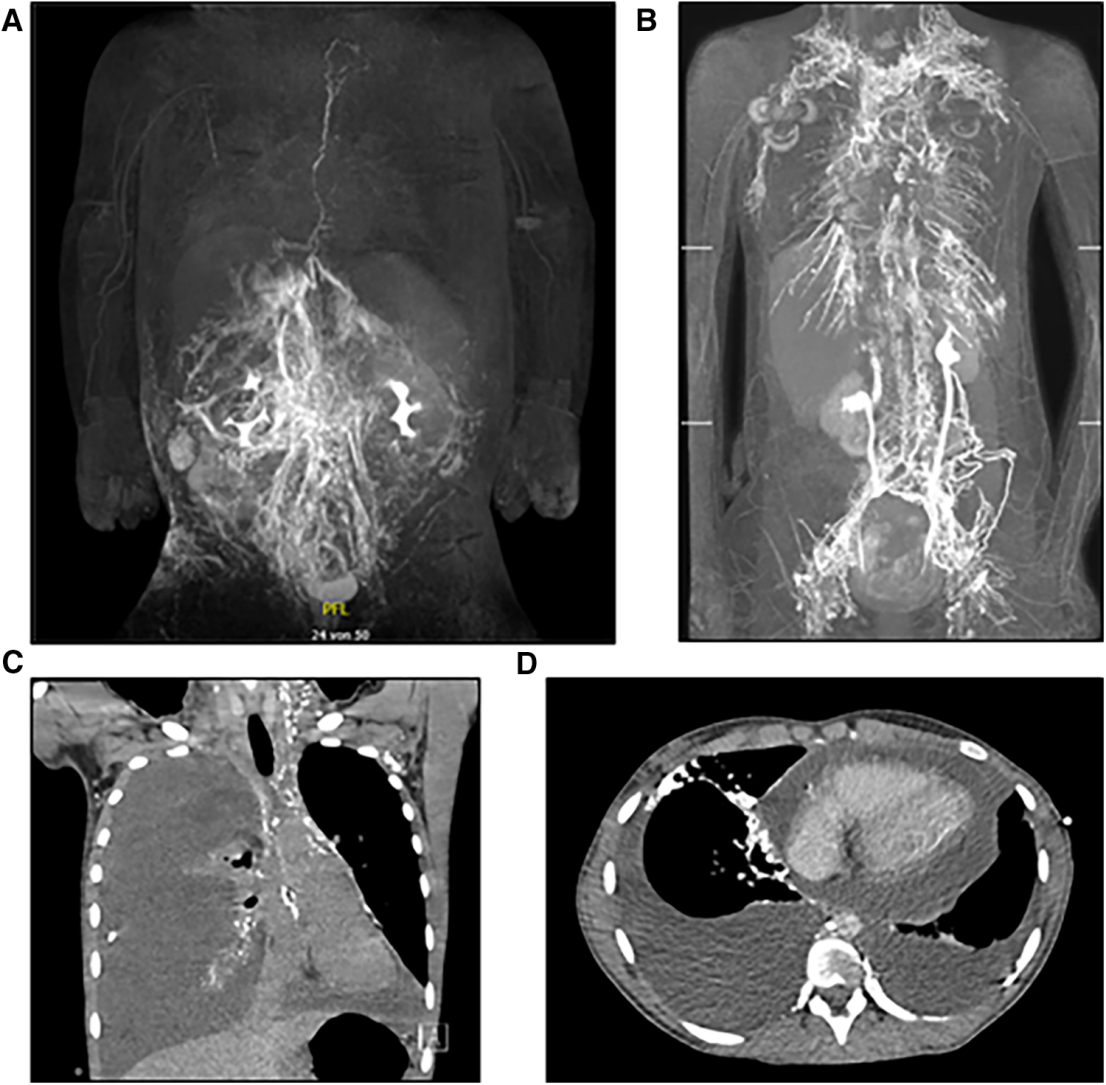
(A) MR-lymphangiography of a thrombotic occlusion at the left venous angle in a 6-month-old female patient as an example of an acquired central lymphatic flow disorder. (B) MR-lymphangiography of a 19-year-old patient with significant reflux in the context of a lymphatic drainage disorder, the congenital lesion is caused by a 13q deletion. (C) Exemplary CT-image of a recurrent chylopericardium. (D) Exemplary CT-image of a recurrent bilateral chylothorax.
